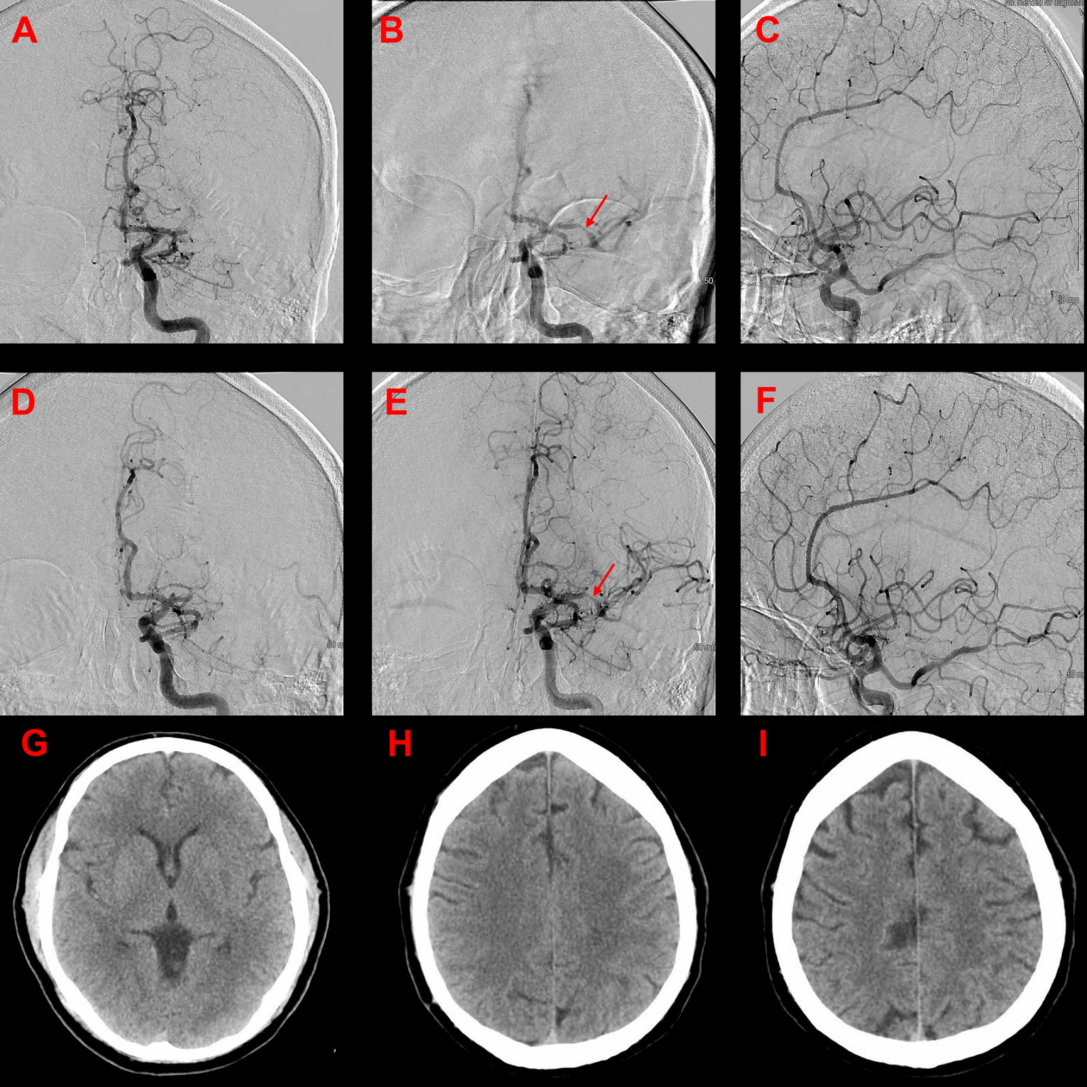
Angiogram of case 2 Initial pre-intervention AP (A) image demonstrating a complete left M1 segment occlusion. Post-stent retriever treatment (B, C), there is restored antegrade flow, but stenosis (arrow) is noted on the AP image. Ten minutes post-thrombectomy, M1 was re-occluded (D). After intra-arterial eptifibatide infusion (E, F), there is restored antegrade flow and reduced stenosis (arrow). CT brain performed at 24 hours (G, I) demonstrates ischemic changes in the basal ganglia and corona radiata. AP: anteroposterior; CT: computed tomography
